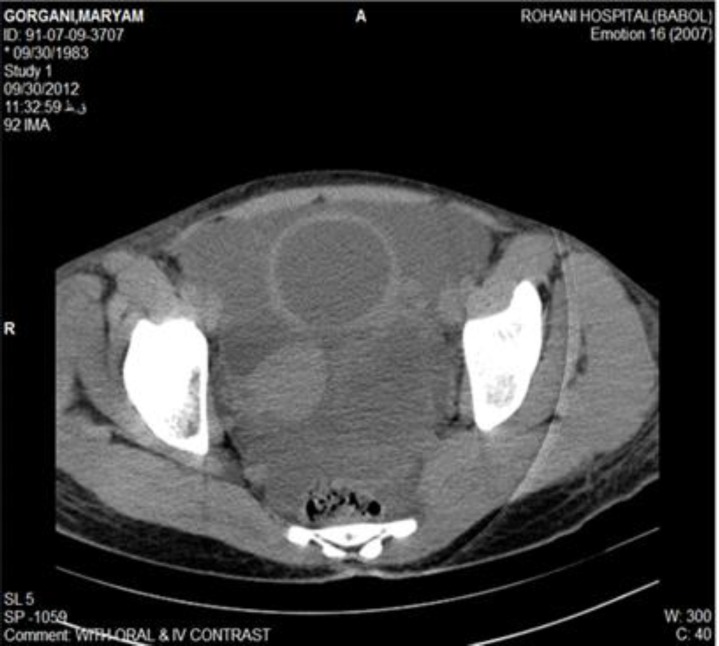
Abdominal CT-scan (with oral and intra-venous contrast
